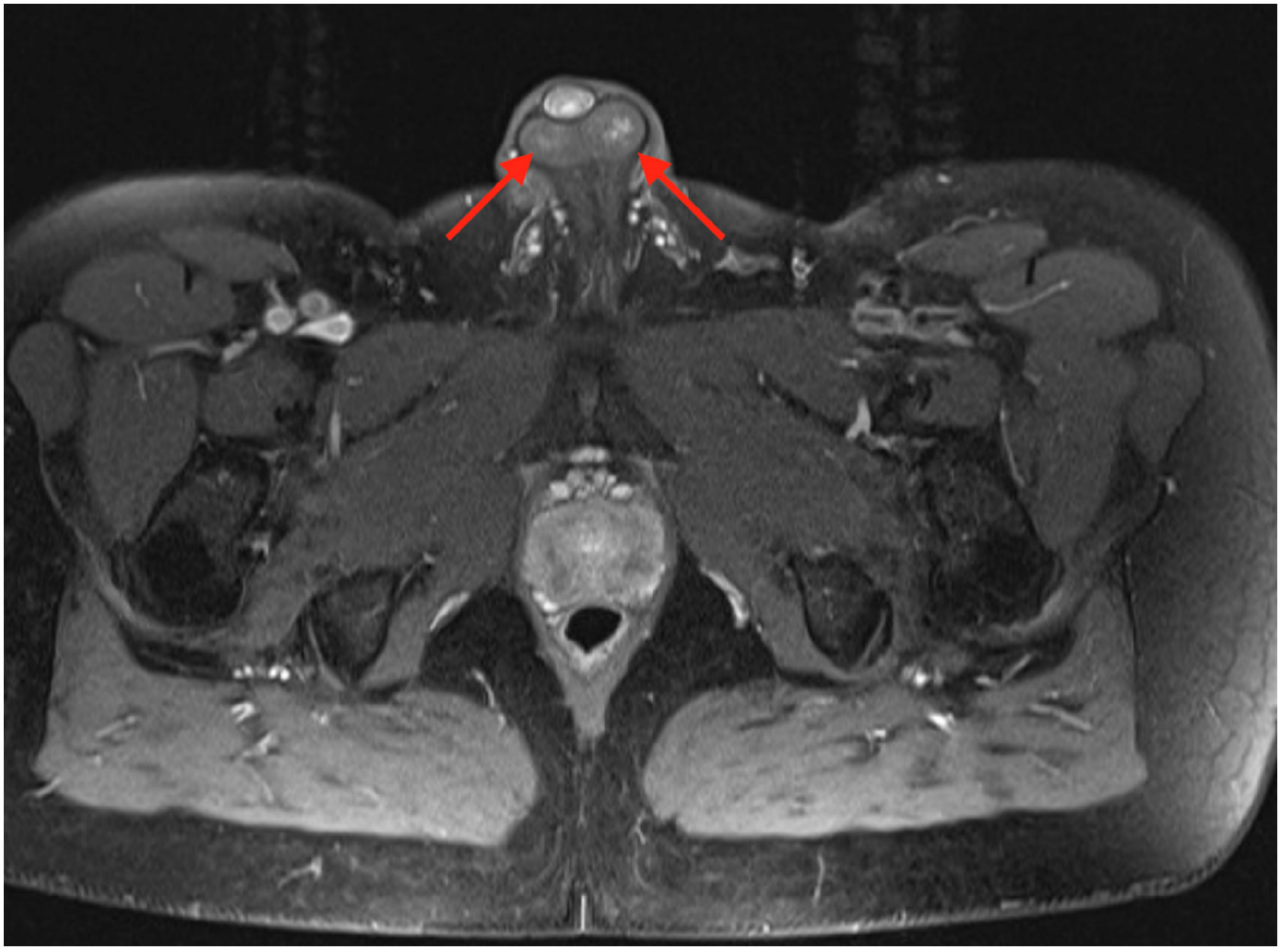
The axial view of scrotal magnetic resonance imaging: the red arrows show a bilateral intratesticular lesion compatible with an adrenal intra testicular inclusion
